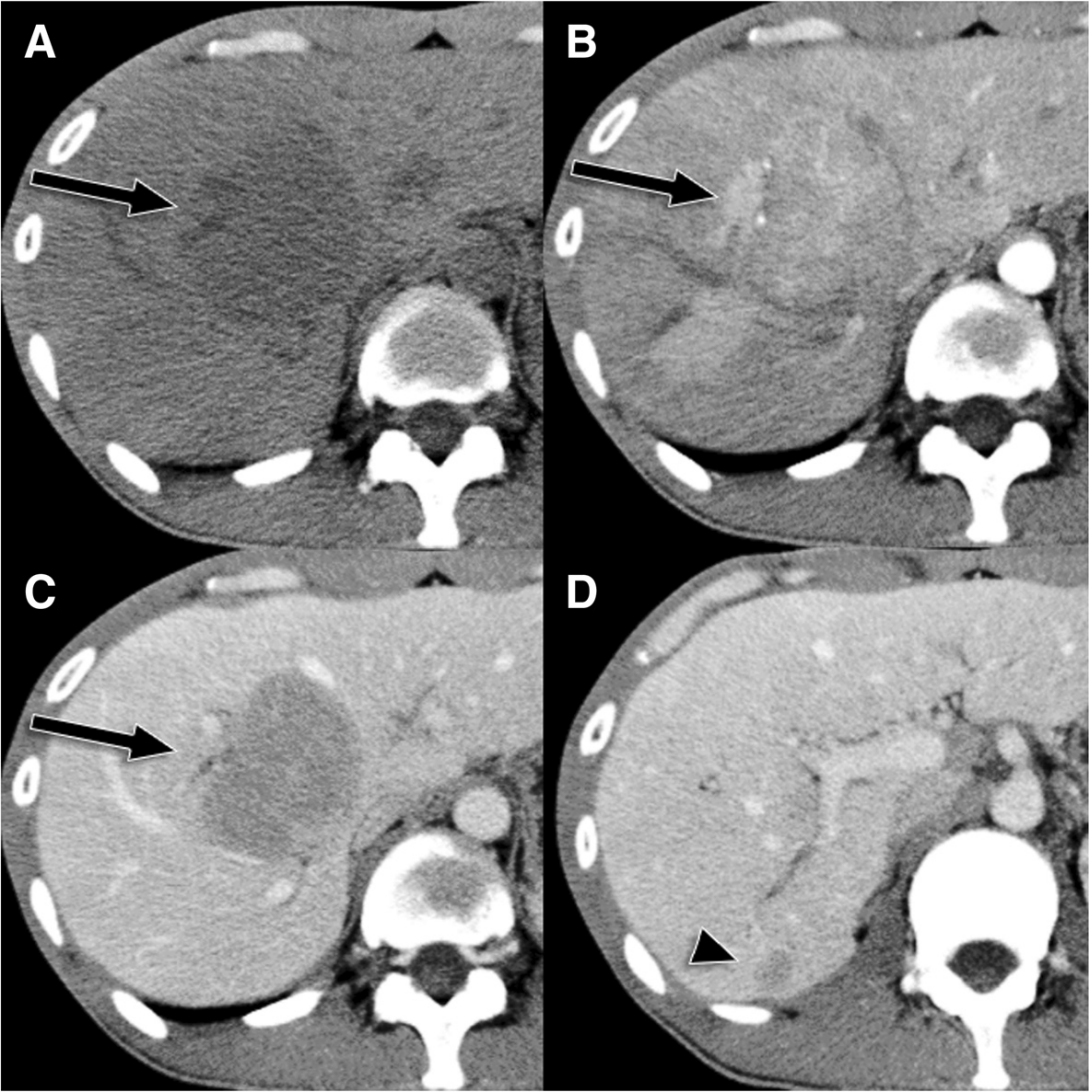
Abdominal computed tomography. (A) Axial computed tomography (CT) imaging shows a liver lesion (arrow) with low attenuation. (B) On early-phase axial contrast-enhanced CT imaging, the lesion exhibits heterogeneous enhancement. (C) On portal-phase axial contrast-enhanced CT imaging, the lesion shows rapid washout in segment 5. (D) The segment 6 lesion exhibits a similar pattern (arrowhead).
